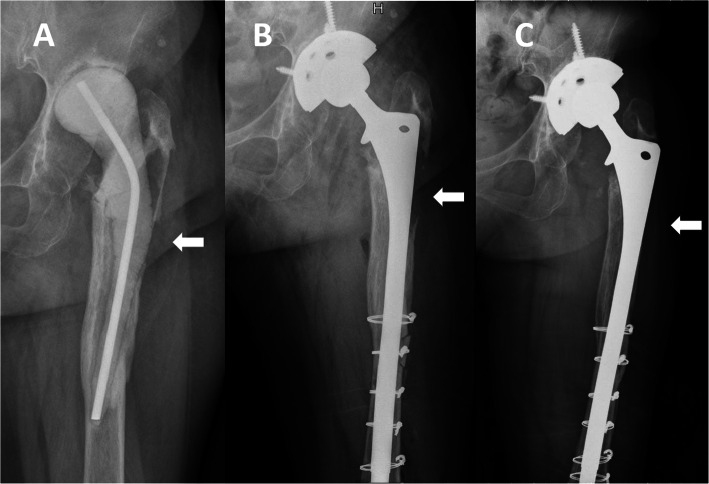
Anteroposterior radiographs of a 76-year-old man who underwent revision THA for a periprosthetic joint infection using extensively porous-coated stem alone. a Radiograph prior to stage 2 revision THA showing a Paprosky type IIIB femoral bone defect. b Postoperative radiograph at 6 months showing no bone restoration in the bone defect area (white arrow). c Postoperative radiograph at 5 years showing bone defects still existing and moderate stress shielding on both the medial and lateral sides
